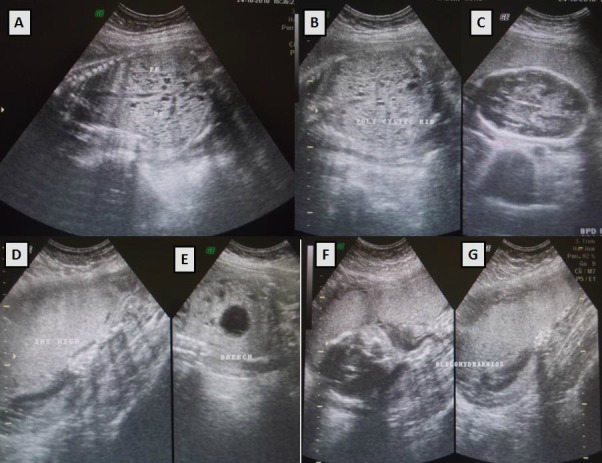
Figure 1: High resolution fetal ultrasonogram depicting (A , B, C) Hyperechoic and enlarged kidneys characteristic of in autosomal recessive polycystic kidney disease; (D) High, anterior placenta; (E) Breech presentation; (F, G)Oligohydramnios
